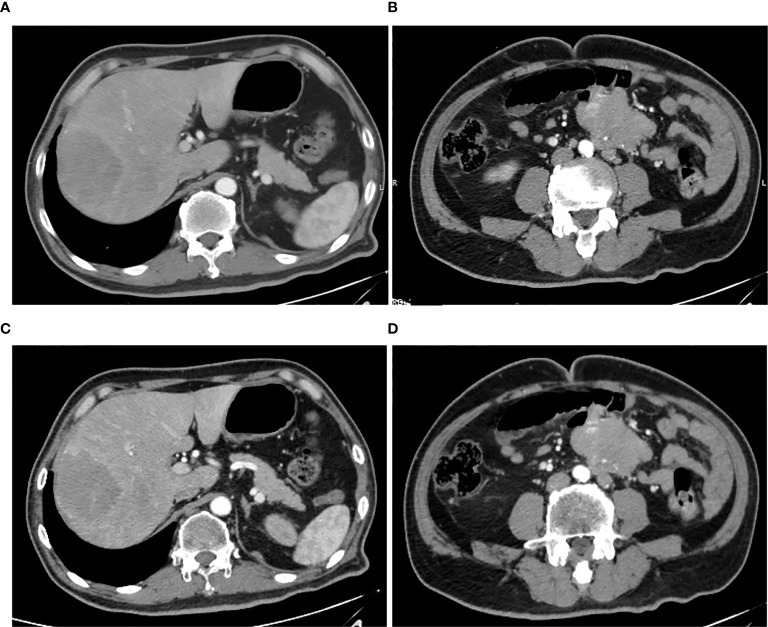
Pretreatment CT images. (A, B) CT arterial and portal phase images of liver metastasis with a size of 70 mm × 61 mm, respectively. (C, D) CT arterial and portal phase images of abdominal & pelvic cavity metastasis with a size of 76 mm × 50 mm, respectively.
